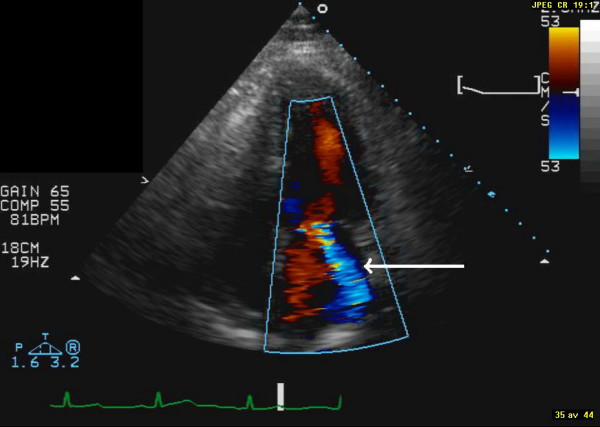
Display of end-systolic image from apical 4-chamber view after six month of treatment with pioglitazone. The white, thicker, arrow indicates the newly developed moderate mitral regurgitation.
